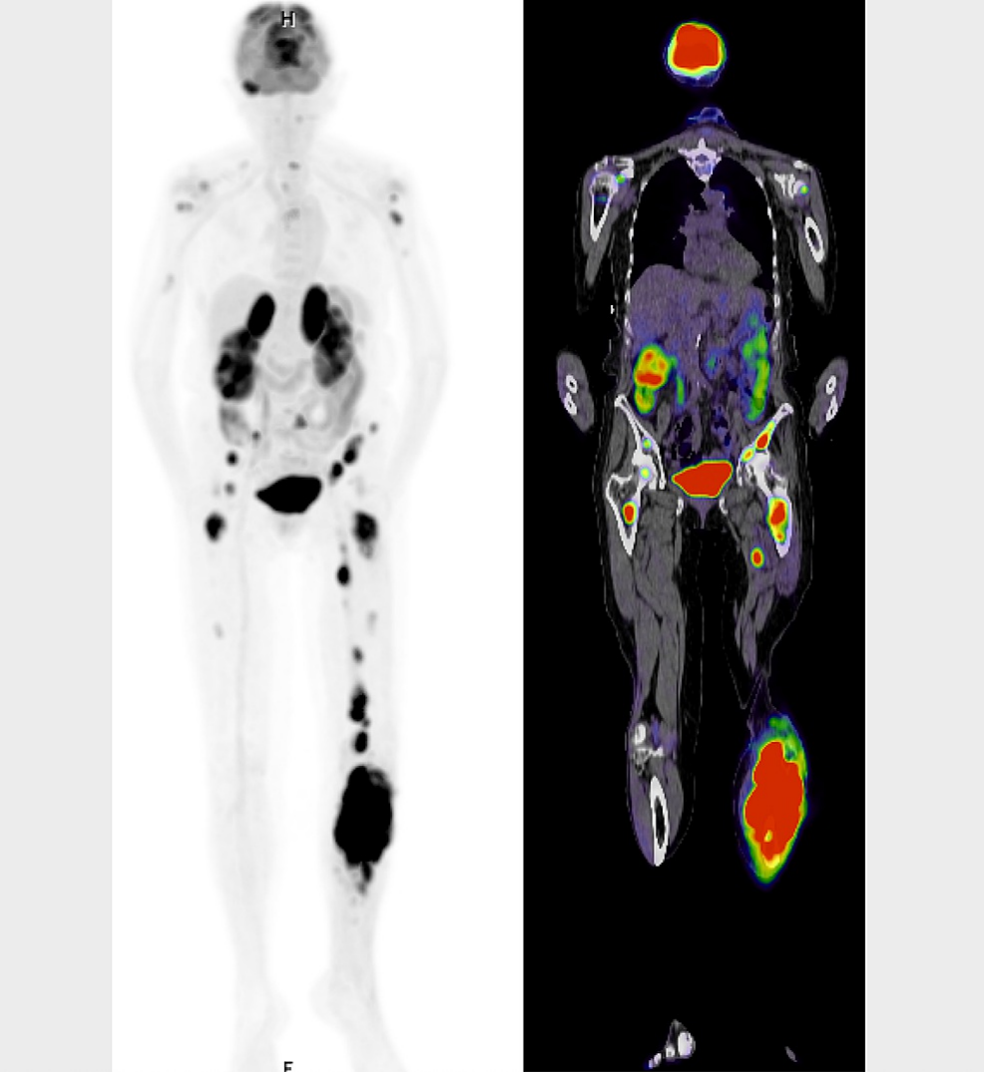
Positron emission tomography-computed tomography (PET-CT) scan demonstrating increased uptake in many areas, most notably the left tibia, which had a maximal standard uptake value (SUV) of 22.38. Other areas with high SUV values included the left proximal humerus (SUV 6.96), right temporal bone (SUV 9.41), left acetabulum (SUV 13.62), left adrenal gland (SUV 14.4), and the left adductor musculature (SUV 17.37). There is also multifocal lymphomatous involvement of the axial and appendicular skeleton.
